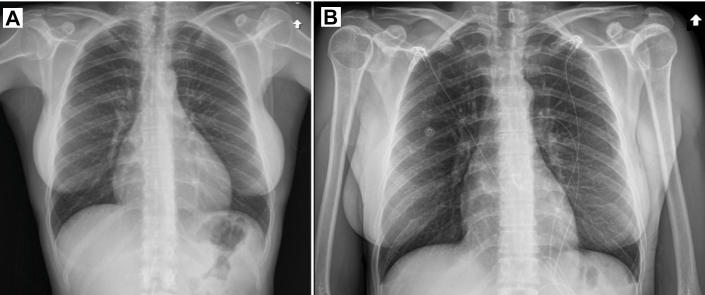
Chest Radiographs Before and After Pericardiocentesis Upright anteroposterior chest radiographs (A) before and (B) after pericardiocentesis. Compared with the initial study, the postprocedural image demonstrates a reduction in the cardiopericardial silhouette after removal of the pericardial drain.
